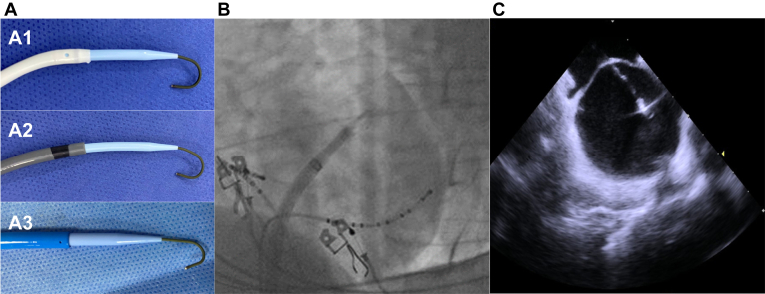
The tip of the guidewire regains its J-shape upon crossing the septum. A: Sheaths with the guidewire regaining the J-shape: nondeflectable sheath (A1), deflectable sheath (A2), cryoballoon sheath (A3). B: Fluoroscopy view of the guidewire crossing the septum regaining its J-shape. C: Intracardiac echocardiography showing the guidewire crossing the septum regaining its J-shape.
